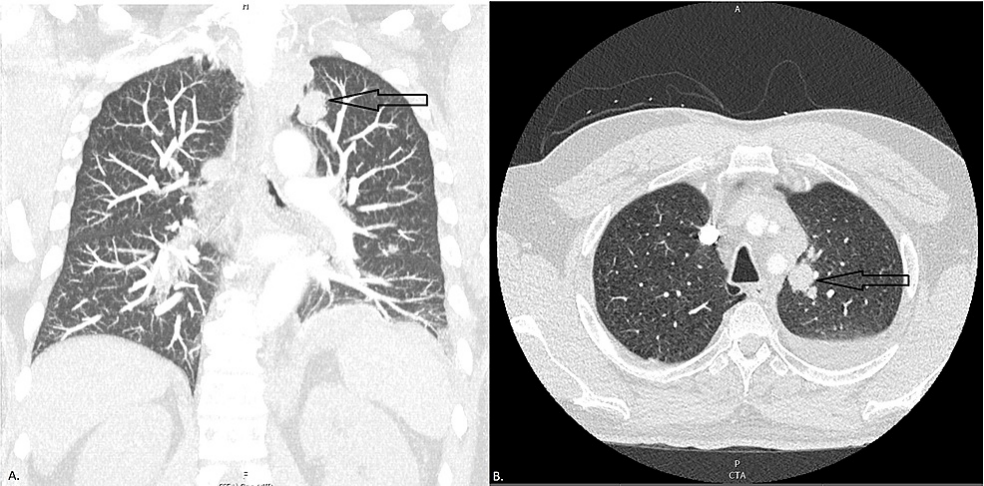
Computed tomography of the thorax with contrast at the time of diagnosis with lung cancer (A) Coronal and (B) axial views demonstrate a large 2.3 × 1.4 cm^2^ nodule in the medial aspect of the left upper lobe suspicious for primary lung cancer (black arrows) with a moderate-sized left pleural effusion and compressive atelectasis in the left lung base.
